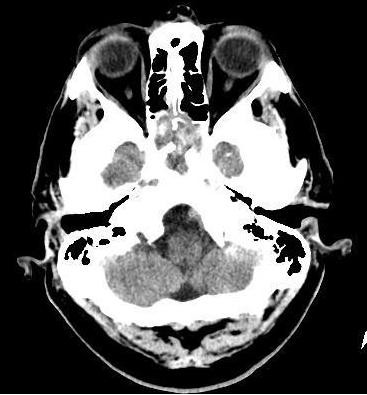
CT Scan on admission showing opacification of the sphenoid sinuses.
